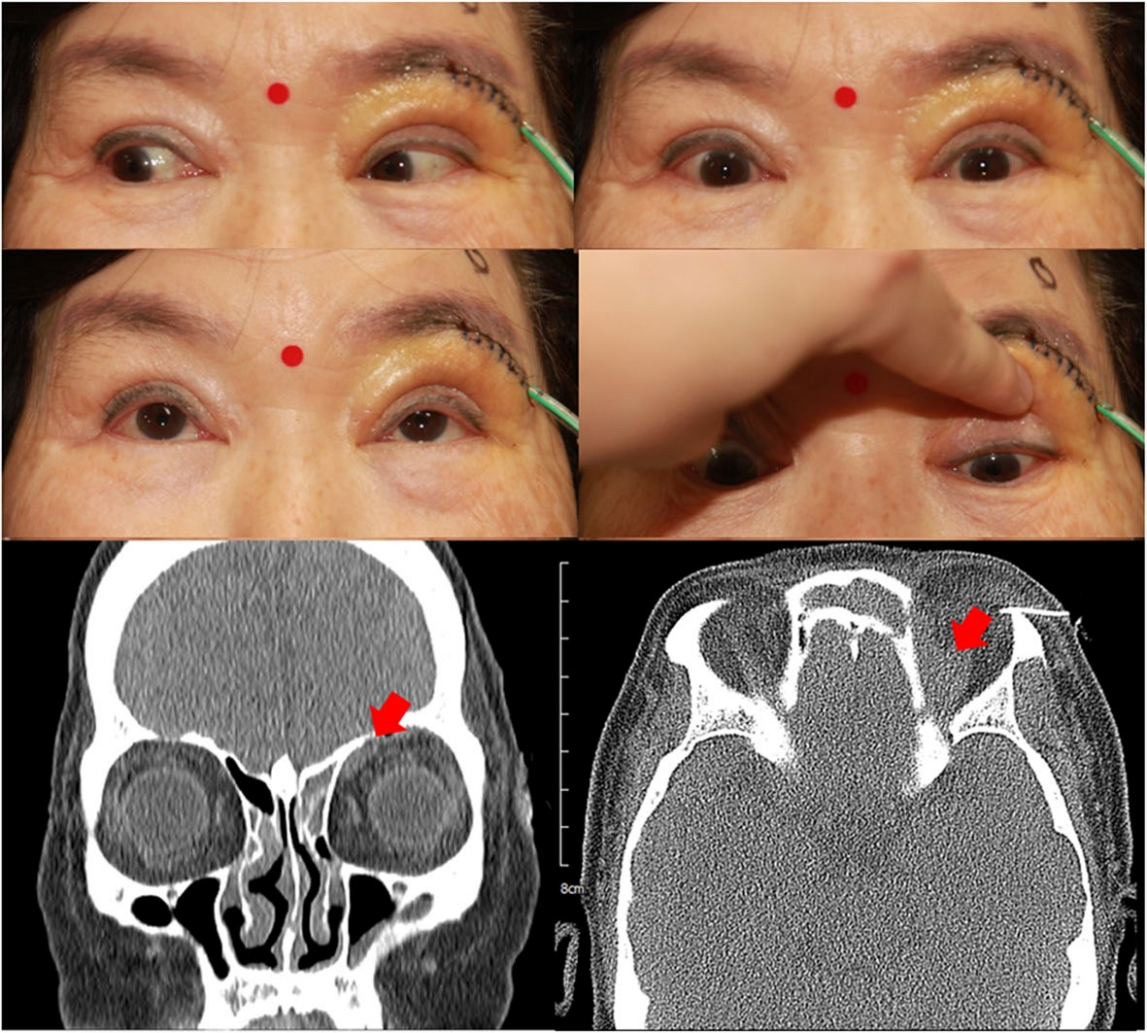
Photograph of the patient, postoperative day 3. Extraocular muscle movement in elevation, depression and adduction of the left eye is normal. Orbital subperiosteal hematoma has decreased in size on non-enhanced CT, postoperative day 4
